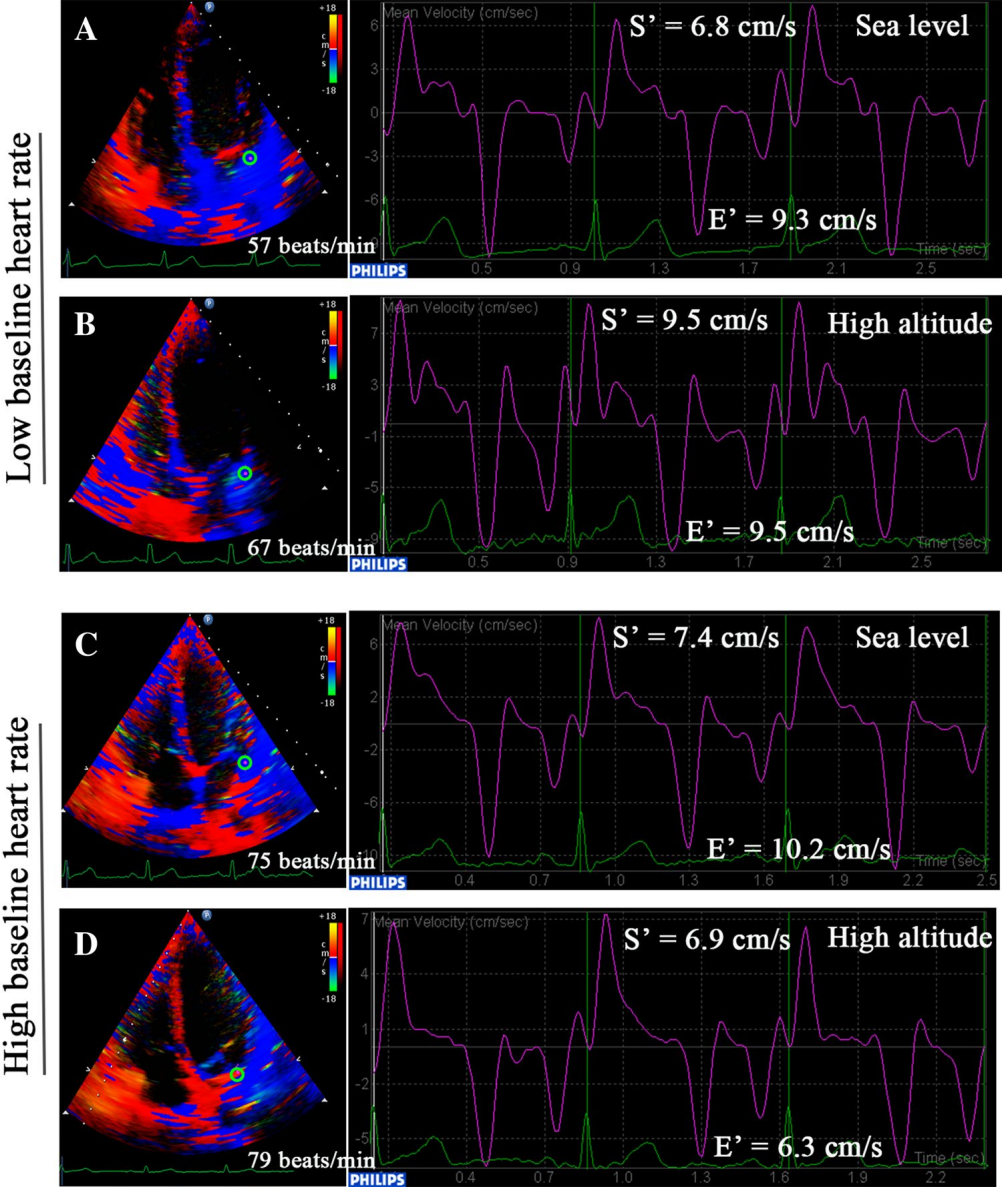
Color tissue Doppler imaging and the curves. The systolic (S′) positive wave and early diastolic (E′) tissue velocities were measured at the center of the lateral walls from the mitral annulus in subjects with lowest tertile baseline HR at sea level (a) and high altitude (b), with highest tertile baseline HR at sea level (c) and high altitude (d)
